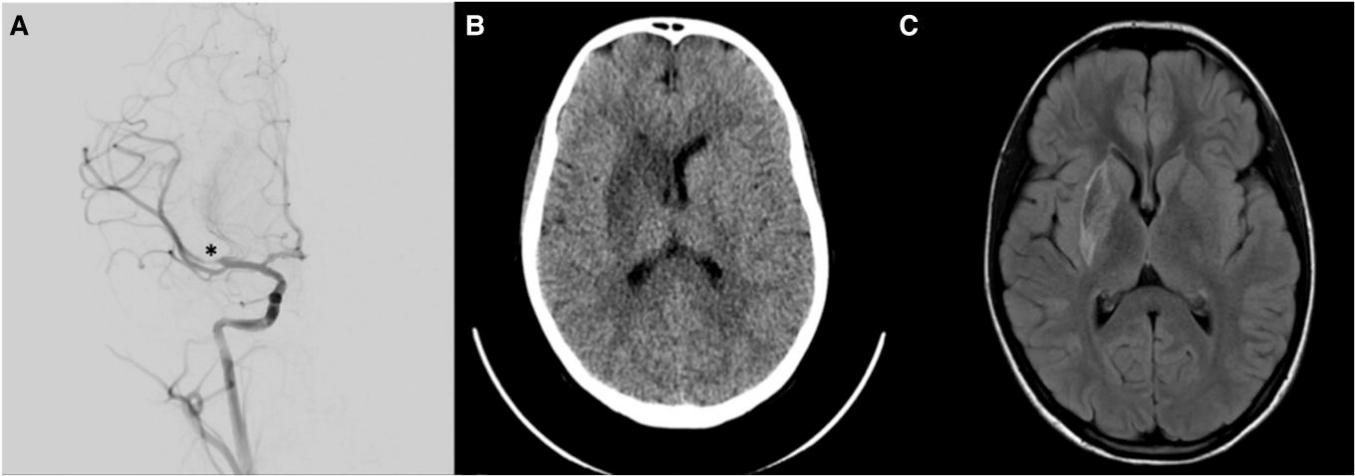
(*A*) Digital subtraction angiography, lateral projection, demonstrates abrupt occlusion of the M1 segment of the right middle cerebral artery (marked with *). (*B*) Non-contrast axial head computed tomography shows a region of hypoattenuation involving the right basal ganglia, as well as the anterior and posterior limbs of the internal capsule. Subtle loss of grey–white matter differentiation is seen in the affected regions, and there is early effacement of the ipsilateral anterior horn of the lateral ventricle. (*C*) Axial diffusion-weighted magnetic resonance imaging demonstrates restricted diffusion in the right basal ganglia and internal capsule, correlating with acute ischaemia. There is associated swelling and partial compression of the adjacent frontal horn of the right lateral ventricle, consistent with early mass effect.
